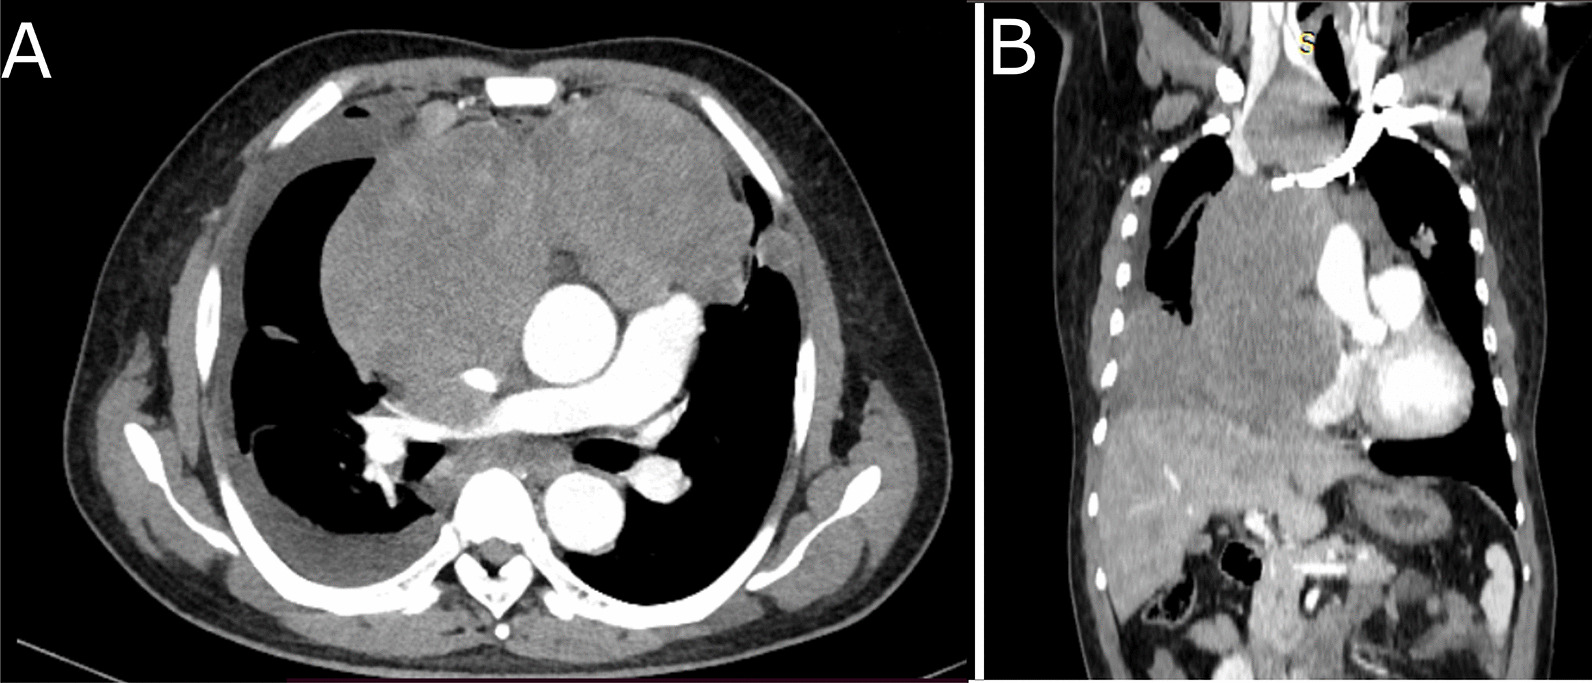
CT scan with injection in axial (A) and coronal (B) sections. Mediastinal lymph node complex and compression of the right superior vena cava
